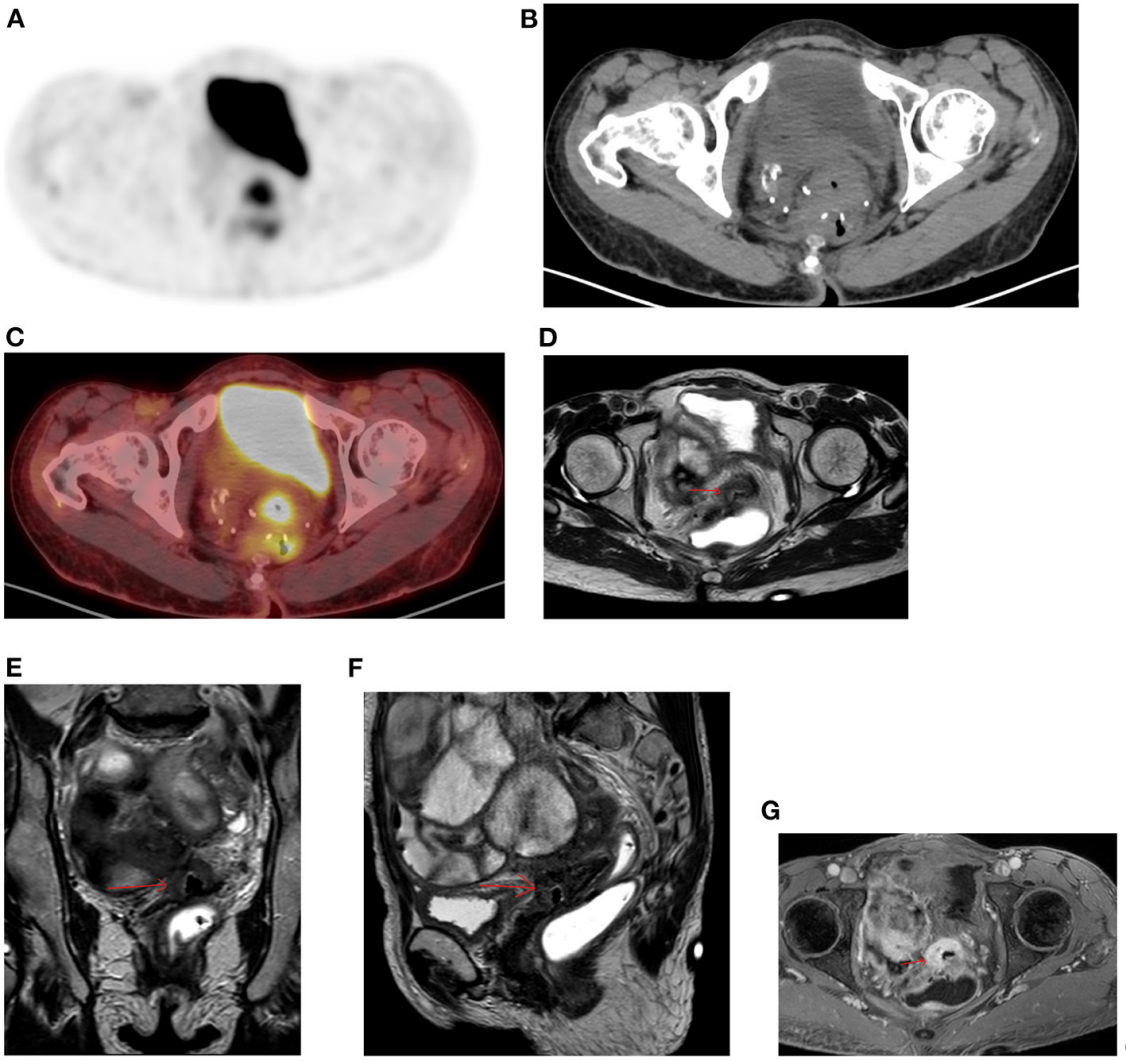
Diffuse uptake in pelvis. FDG PET Axial image (A) demonstrates diffuse increased uptake in central pelvis posterior to bladder that may be consider normal. The corresponding CT (B) showed a soft tissue mass with central air at the suture lines in a 56-year-old female patient with previous history of rectal cancer and had a complete response to chemotherapy. She was referred for restaging due to weight loss and the combined PET/CT Axial (C) image suspected a metastatic lesion despite diffuse uptake. MRI of pelvis was performed, and the Axial, coronal, and sagittal planes (D–F) confirmed the suspicious and showed overt enhancement of the soft tissue mass (G) that is consistent with metastasis.
